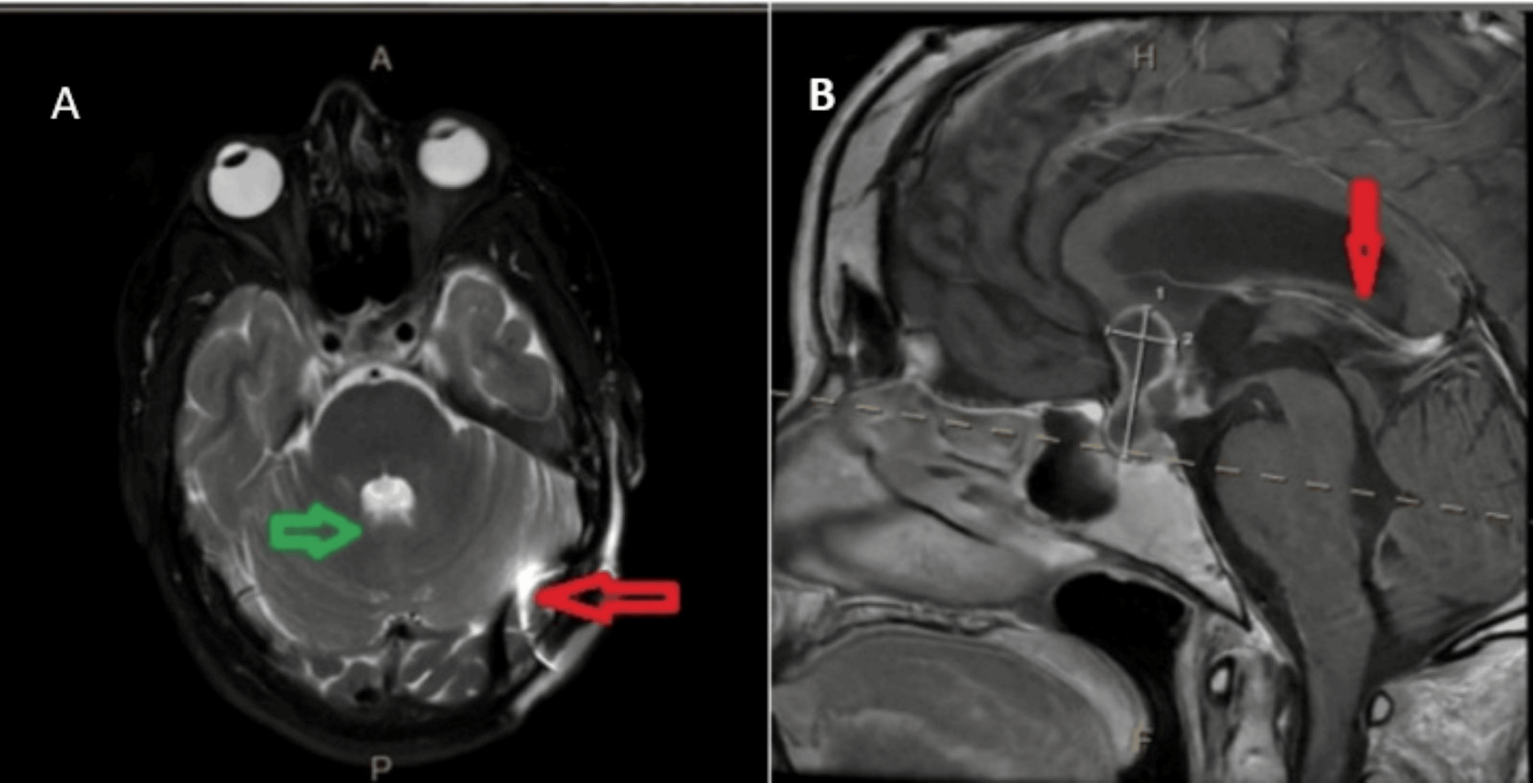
MRI brain with and without contrast A. Axial T2-weighted MRI. B Sagittal section T1 MRI. Green arrow: stable peripherally enhancing cystic sellar/suprasellar lesion consistent with a history of craniopharyngioma. Red arrow: indwelling left occipital ventriculostomy catheter intact, stable size and configuration of the ventricular system MRI: magnetic resonance imaging
